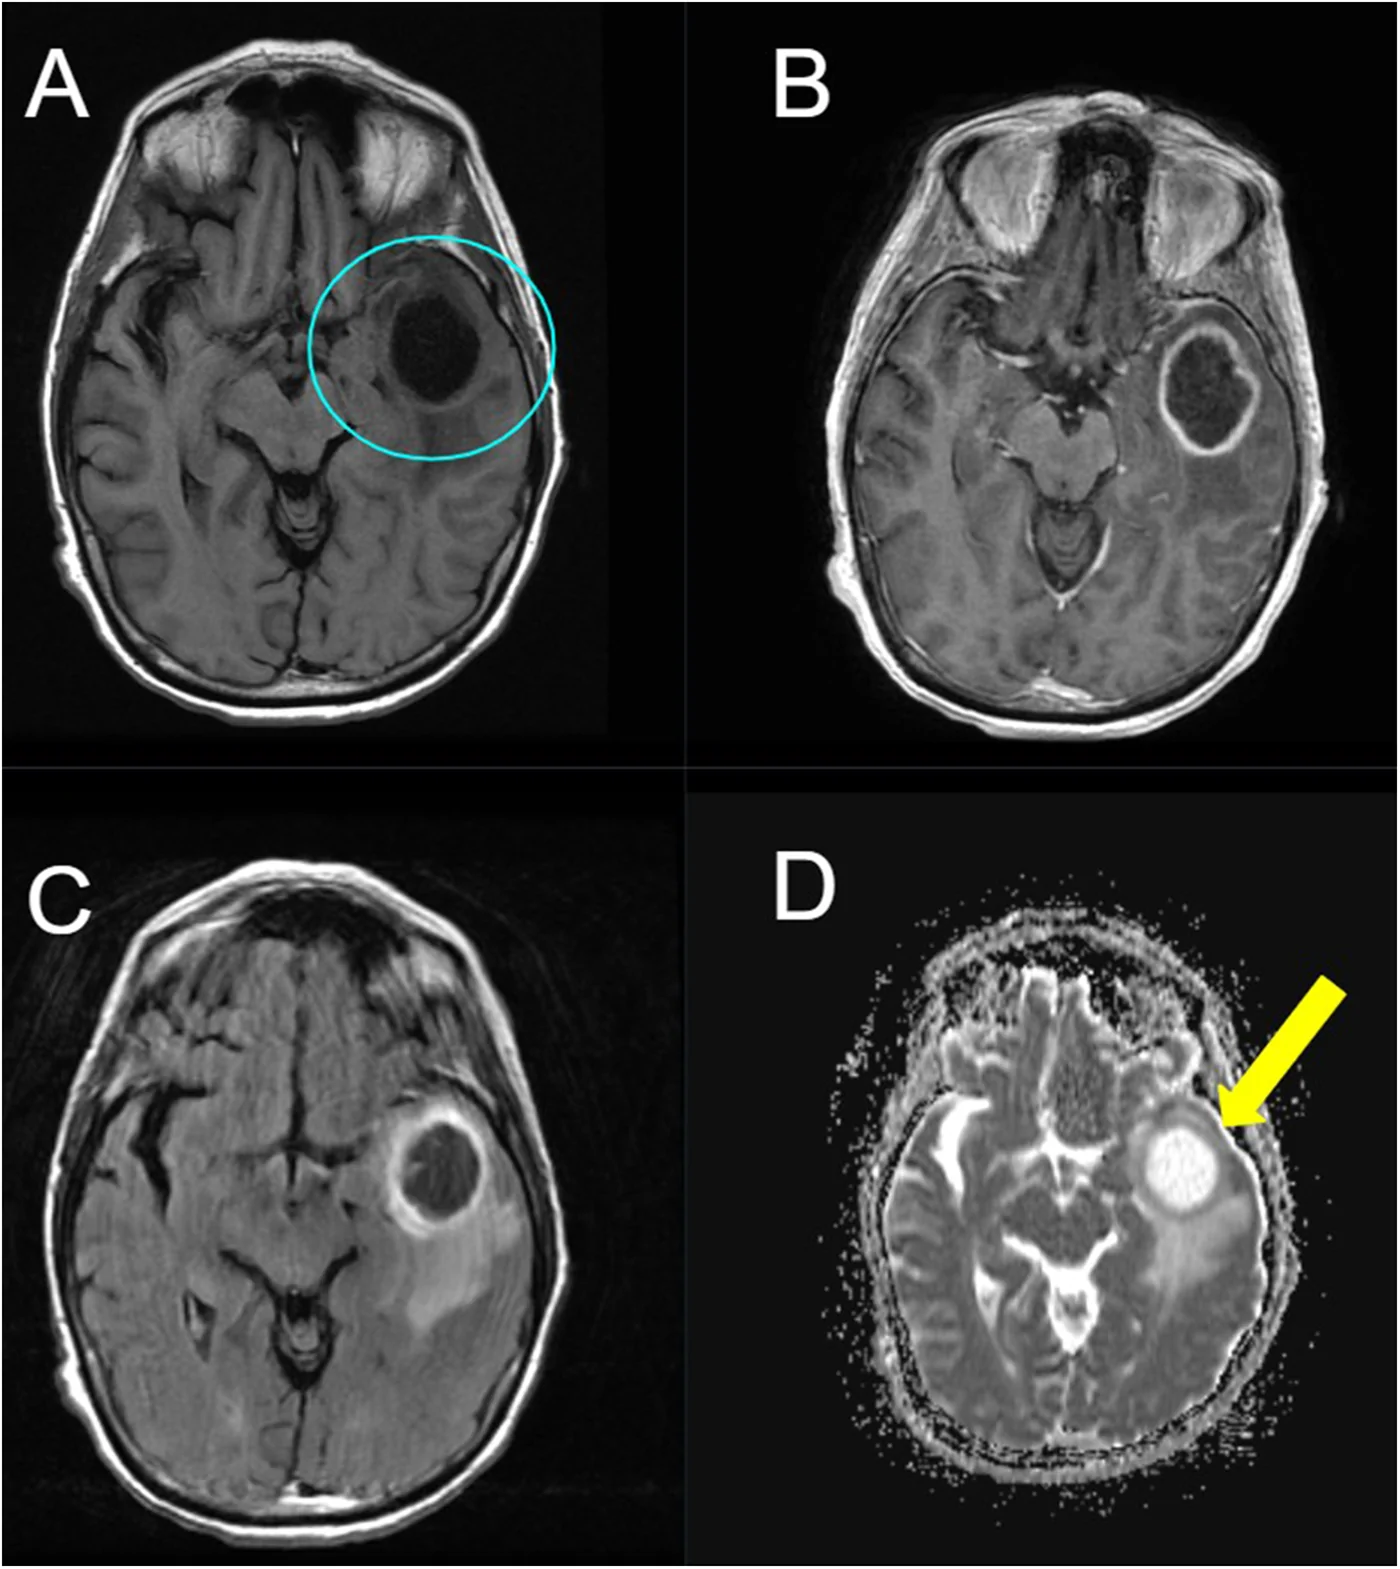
Glioblastoma. (A) Axial T1-weighted pre-contrast MRI shows a centrally T1 hypointense intraaxial mass centered within the left temporal lobe (Blue circle). (B) Axial T1-weighted post-contrast image demonstrates central hypointensity consistent with necrotic/cystic change, surrounded by thick, irregular peripheral ring enhancement. (C) Axial FLAIR image demonstrates associated surrounding FLAIR hyperintense edema. (D) ADC map shows low ADC values along the peripheral rim suggesting high cellularity and a higher-grade lesion. The central necrotic component demonstrates increased ADC values (yellow arrow).
